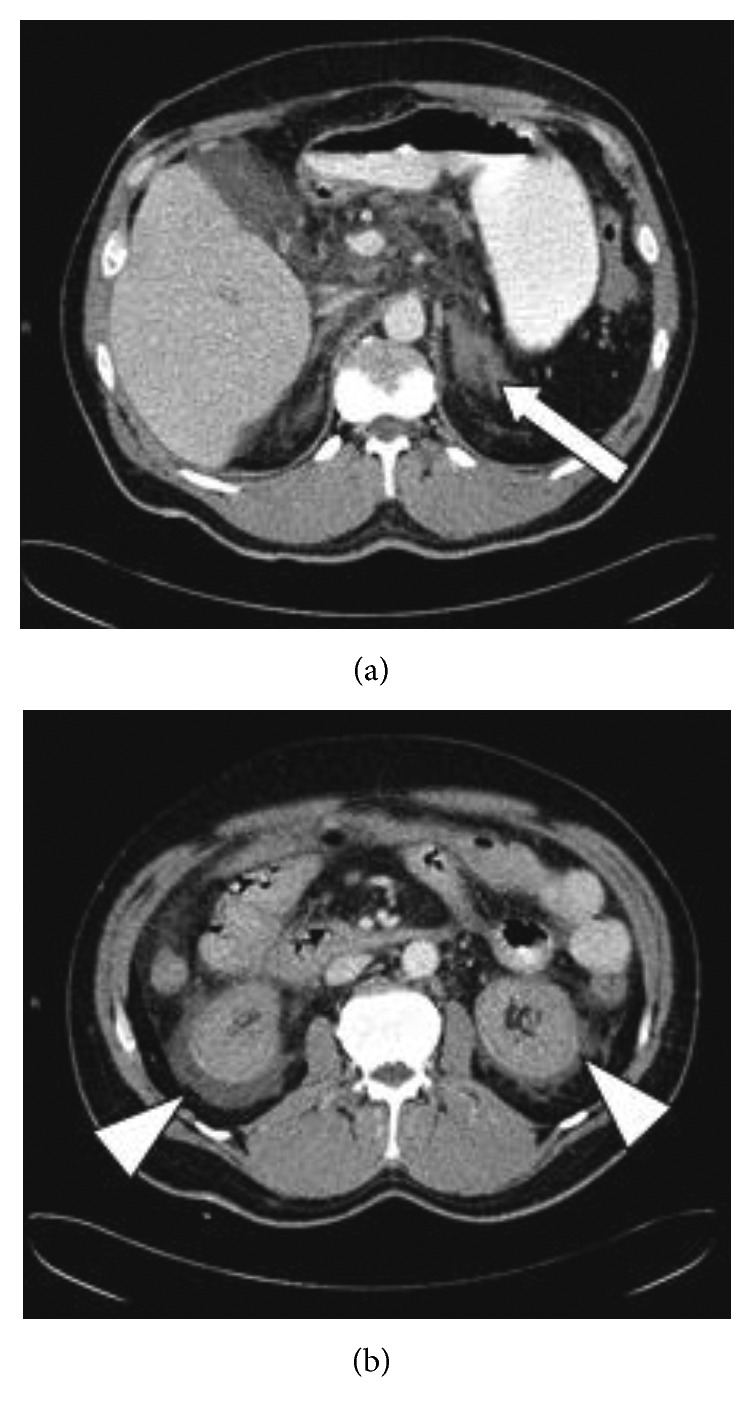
Contrast-enhanced abdominal CT demonstrates haemorrhage of the swollen adrenal glands (arrow) and status after splenectomy (a). There is fluid collection in the perirenal space (arrowheads), and the corticomedullary differentiation of the kidneys is lost (b).
